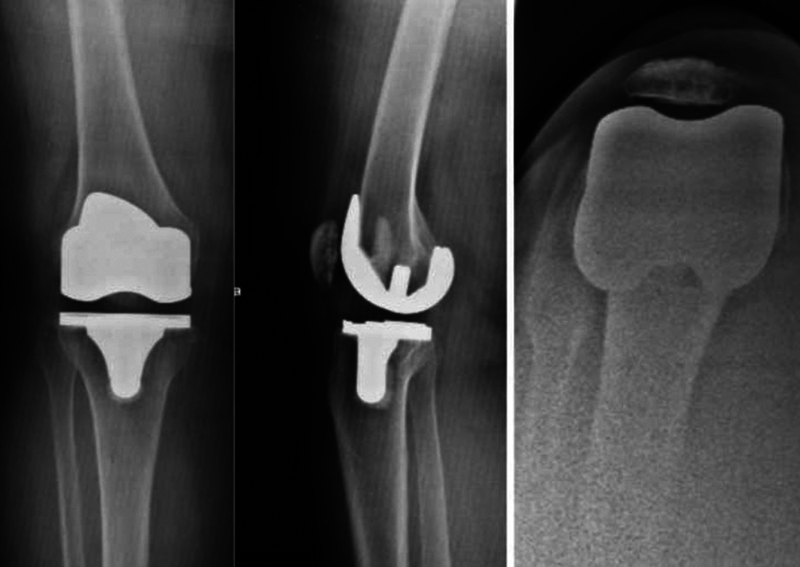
Two years follow-up post reduction radiographs.
